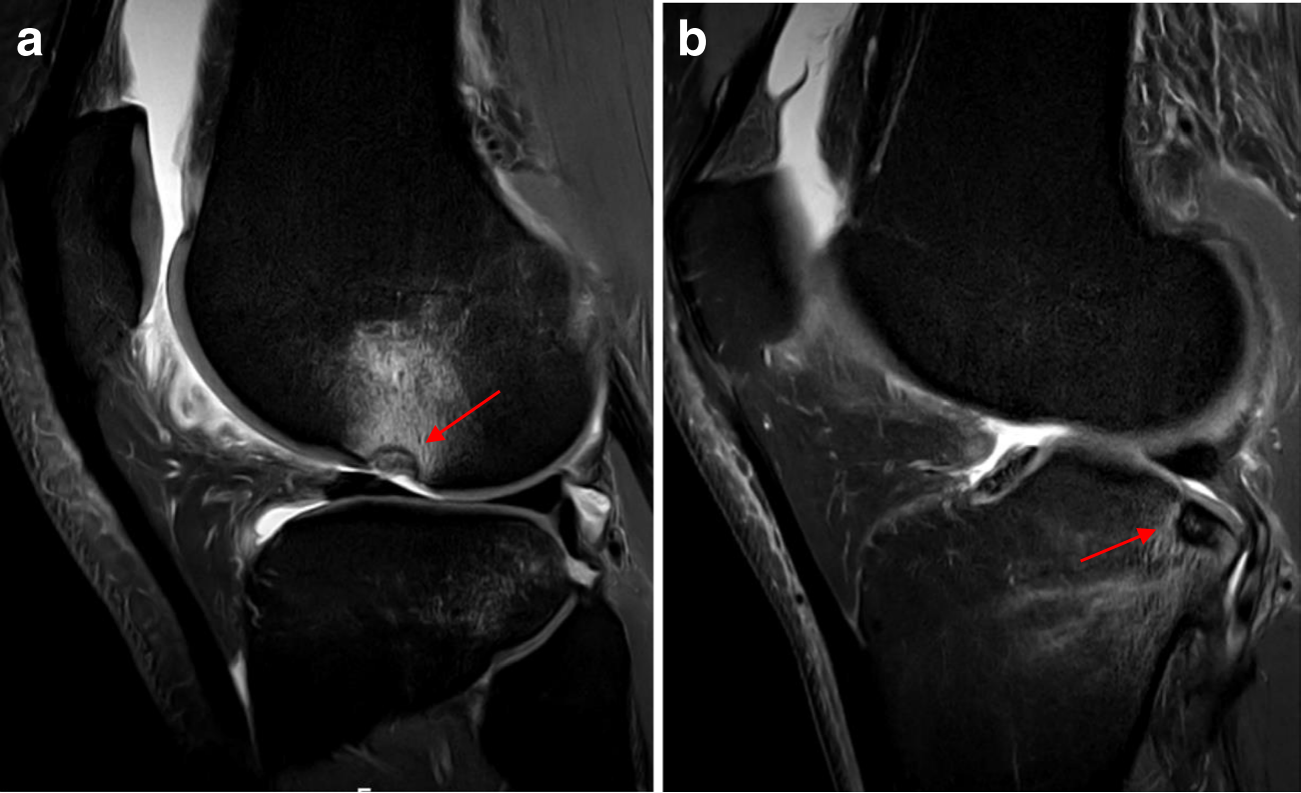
Impaction fracture in the lateral femoral condyle (a) and posterolateral tibia (b) (arrows) on sagittal proton density fat saturation magnetic resonance imaging (right knee)
